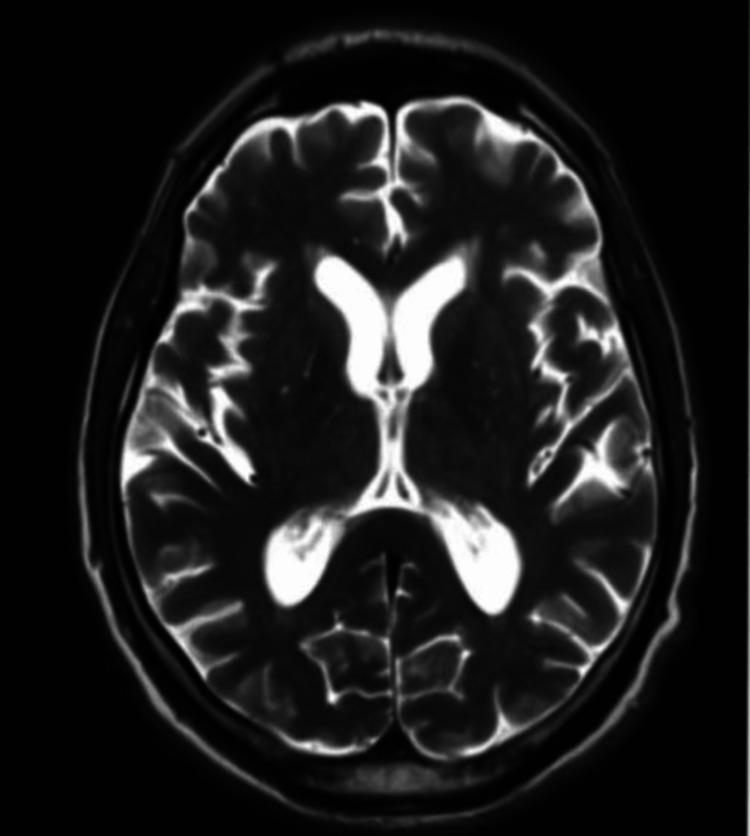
MRI brain: Day-92 axial T2-weighted image showing lesions noted on day 60 have resolved
